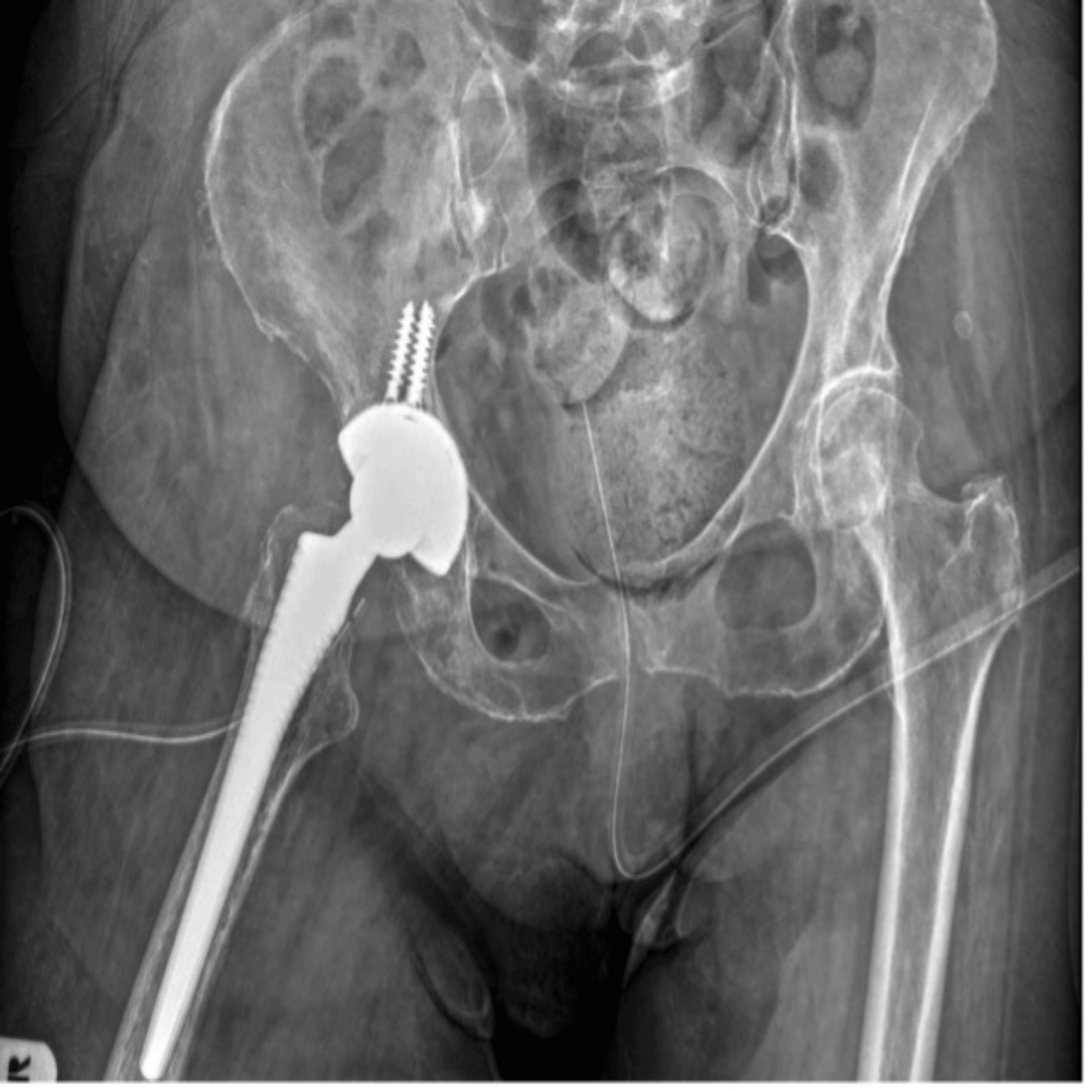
Post-reimplantation plain radiograph
